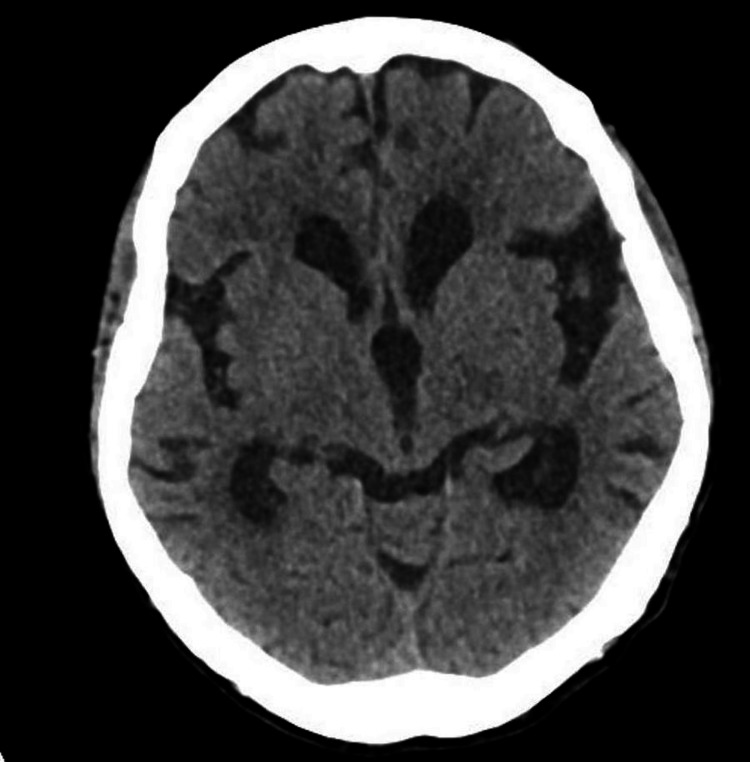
CT head, axial view, no bleeding, no acute changes suggestive of stroke, ASPECT score is 10. ASPECT: Alberta Stroke Program Early CT Score.
